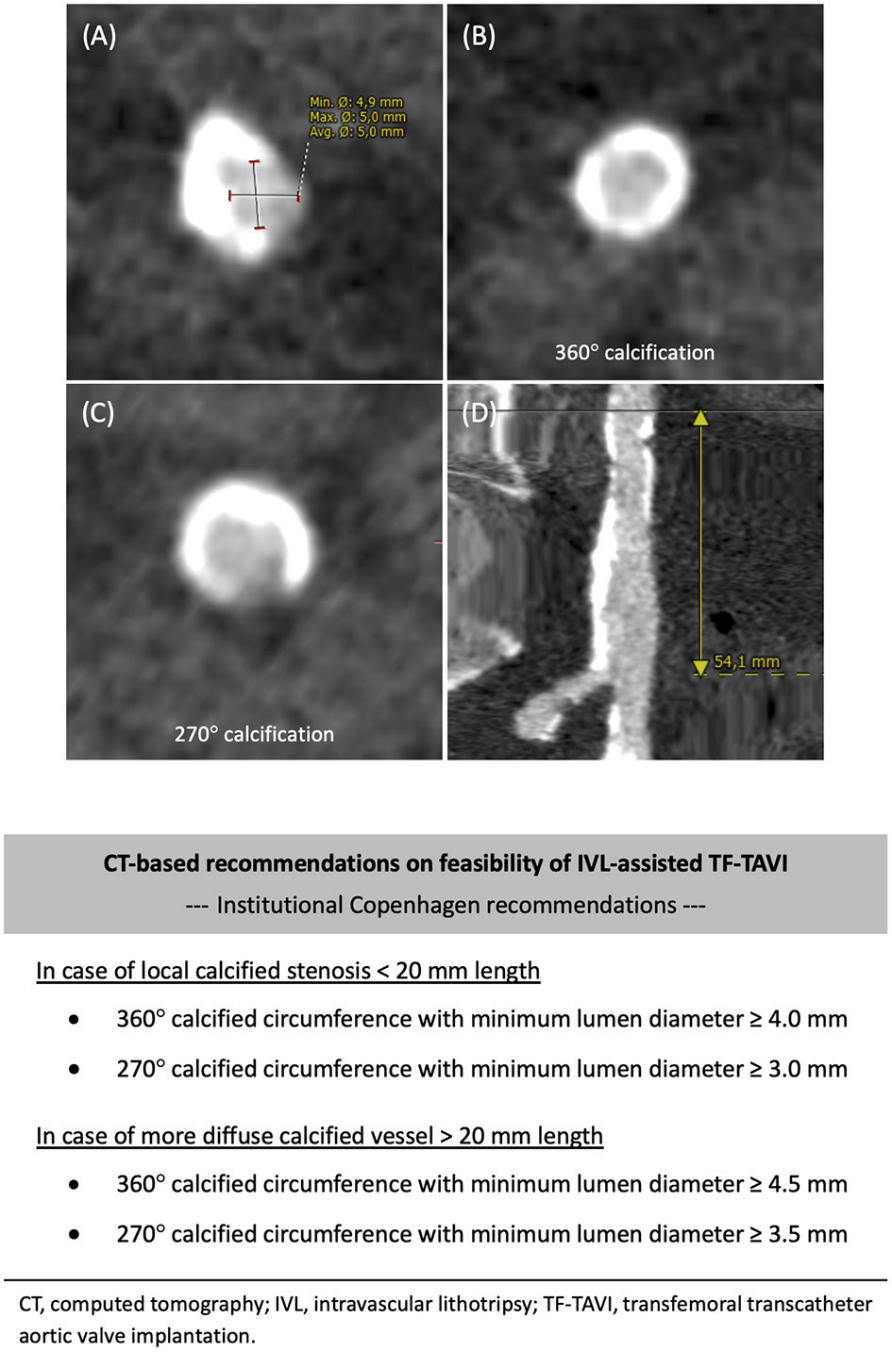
Preprocedural CT analysis. Preprocedural computed tomography (CT) angiography analysis of the iliofemoral access. Standard assessments include: (A) Maximal and minimal diameter measured at the minimal luminal diameter (MLD) of the vessel. (B,C) Circumference of calcification: 360° or horseshoe-like (270°) calcification. (D) Total length of the vessel calcification(s). Copenhagen recommendations on feasibility of IVL-assisted TF-TAVI based on CT angiography analysis. IVL, intravascular lithotripsy; TAVI, transcatheter aortic valve implantation; TF, transfemoral.
